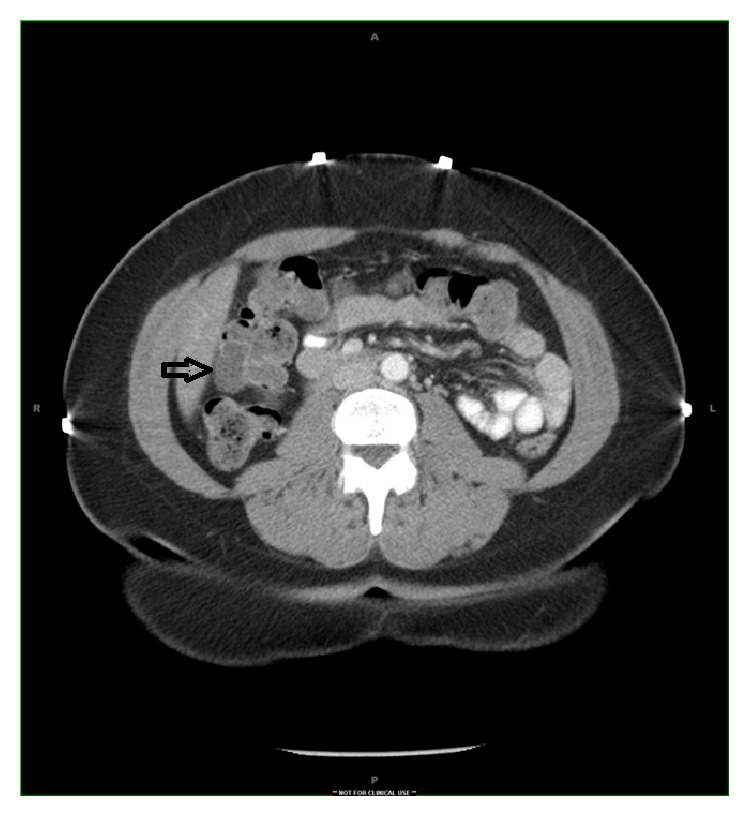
Computed tomographic image showing moderately enhancing cystic lesion at hepatic flexure (arrow).
